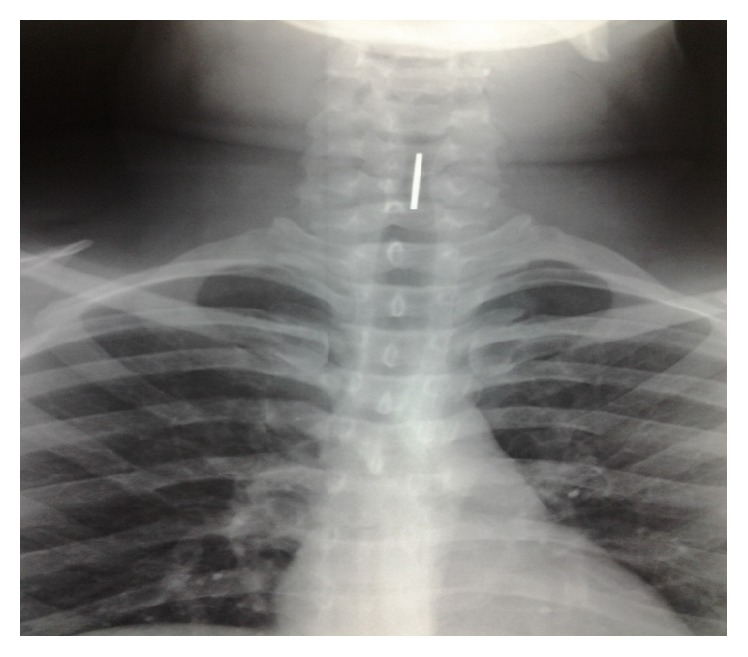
Frontal X-ray: anteroposterior view showing linear opacity from C4 to C5.
